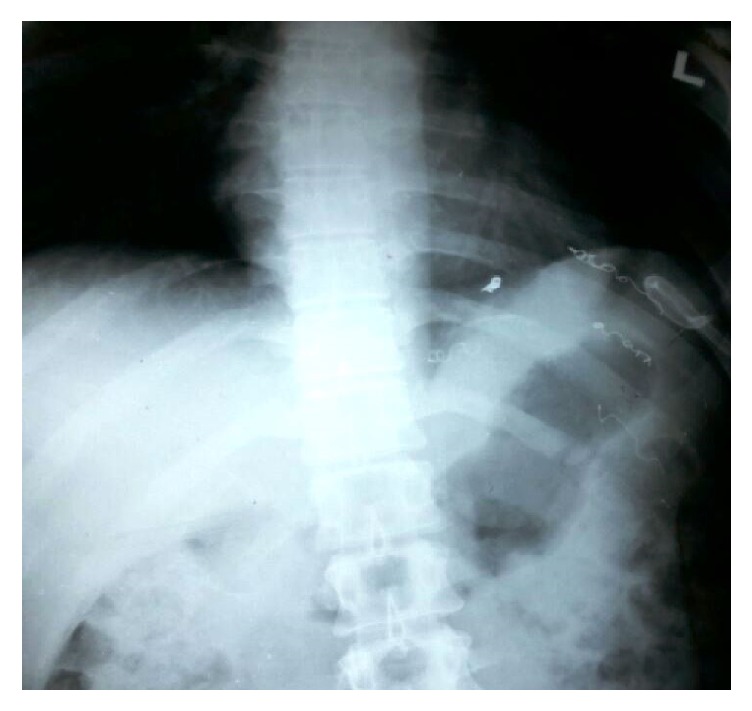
Chest X-ray demonstrating multiple coil embolization done for pseudoaneurysm of left inferior phrenic artery and multiple intercostal arteries. Note the presence of percutaneous placed catheter for drainage of peripancreatic necrosis.
